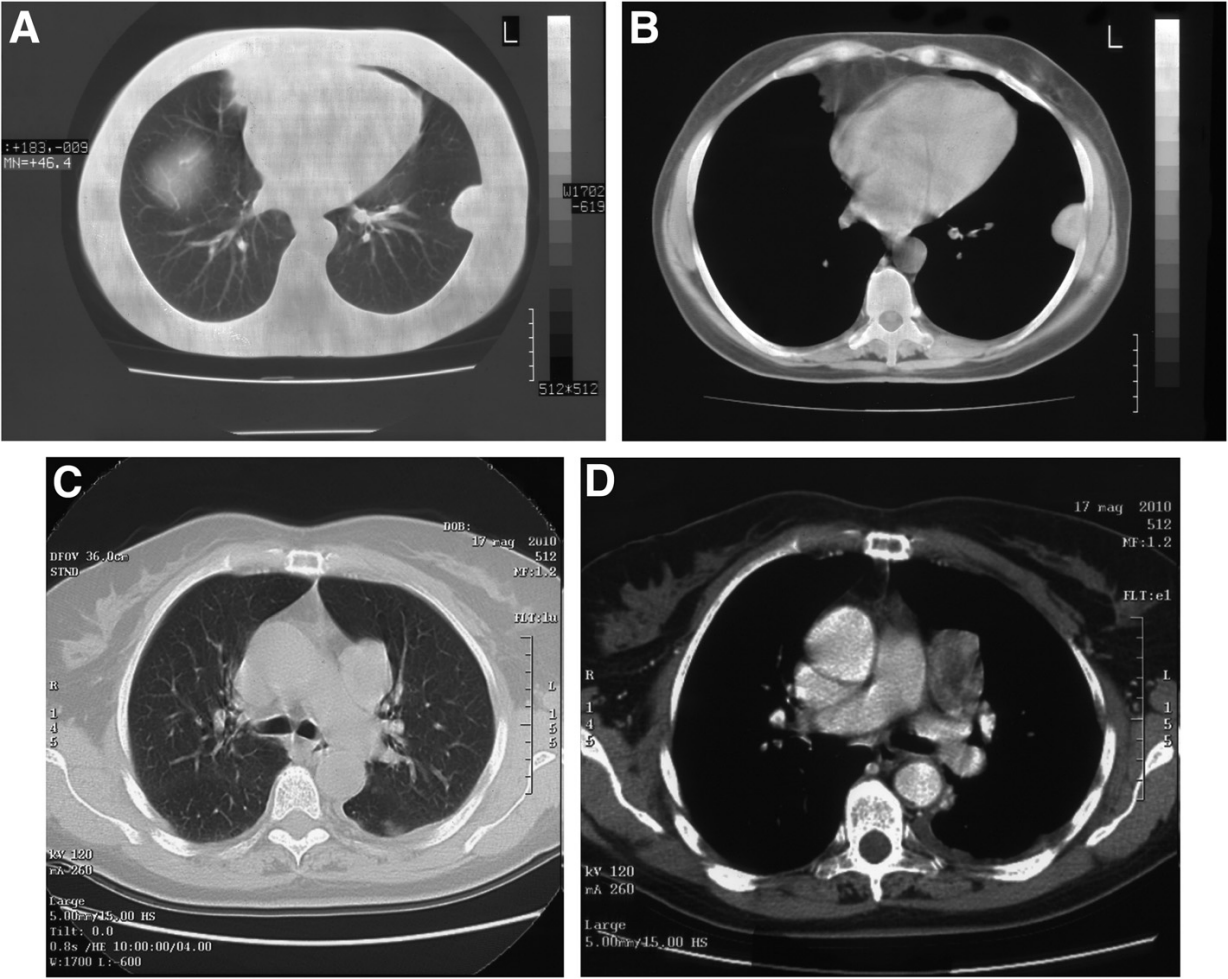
CT scans of primary solitary fibrous tumor of the pleura (1A-B) and the loco-regional relapse in mediastinal pleural (1C-D) after 12 years.
